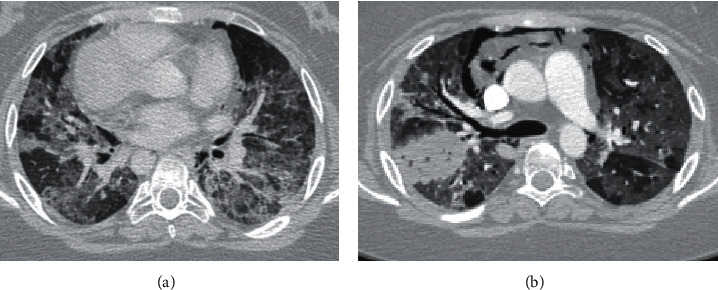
(a) Chest CT scan obtained for case 1, showing diffuse ground glass opacities consistent with COVID-19 pneumonia. (b) Repeat chest CT scan for case 1 5 days later, showing a new right-sided pulmonary infarct, found later to be secondary to mucormycosis angioinvasive fungal infection.
